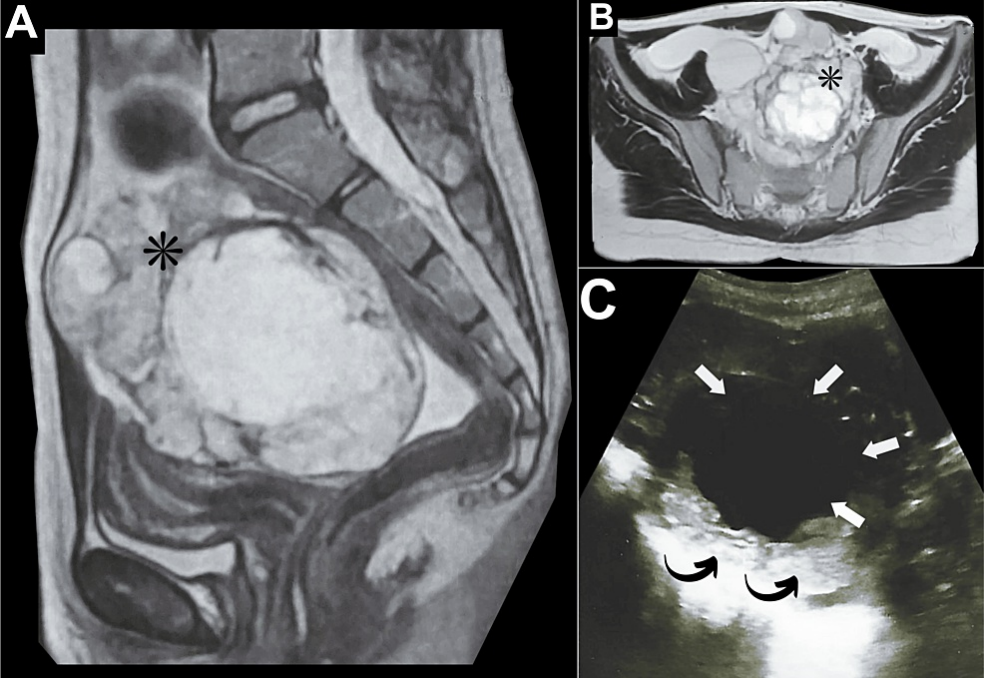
Left ovarian mass (initial diagnostic workup) (A) Initial sagittal T2 pelvic MRI revealed a large ill-defined complex multilocular lesion occupying the left ovary demonstrating contrast enhancement (asterisk). (B) Initial axial T2 MRI revealed a large, ill-defined, complex multilocular lesion (measuring approximately 13 × 8 cm) occupying the left ovary with a thick wall extending to the midline and into the right side after contrast and soft tissue enhancement (asterisk). (C) Initial pelvic US revealed a large left ovarian lesion (measuring approximately 9 × 7 cm) with thickened walls (curved arrows), suggesting a complex left ovarian cyst (arrows).
